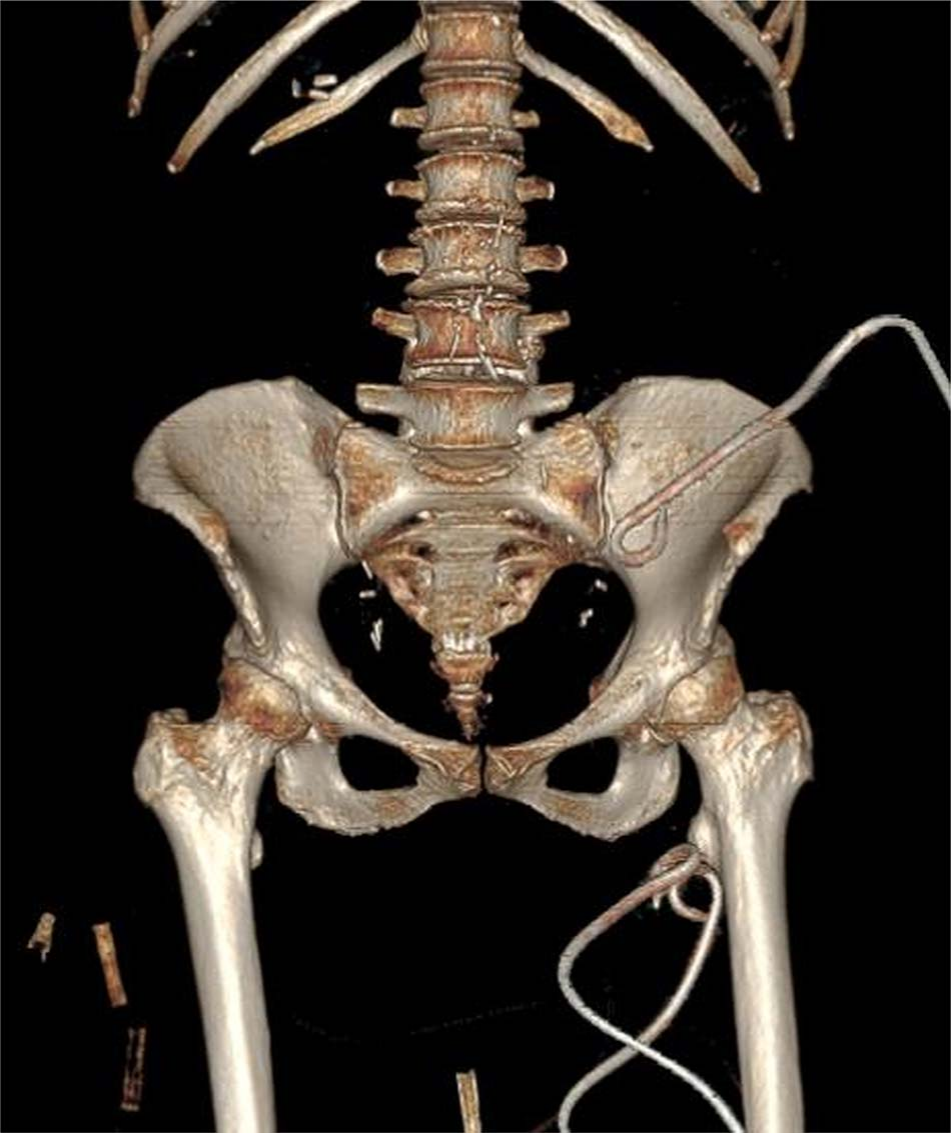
3D CT reconstruction depicting the final positions of the drainage catheters.
